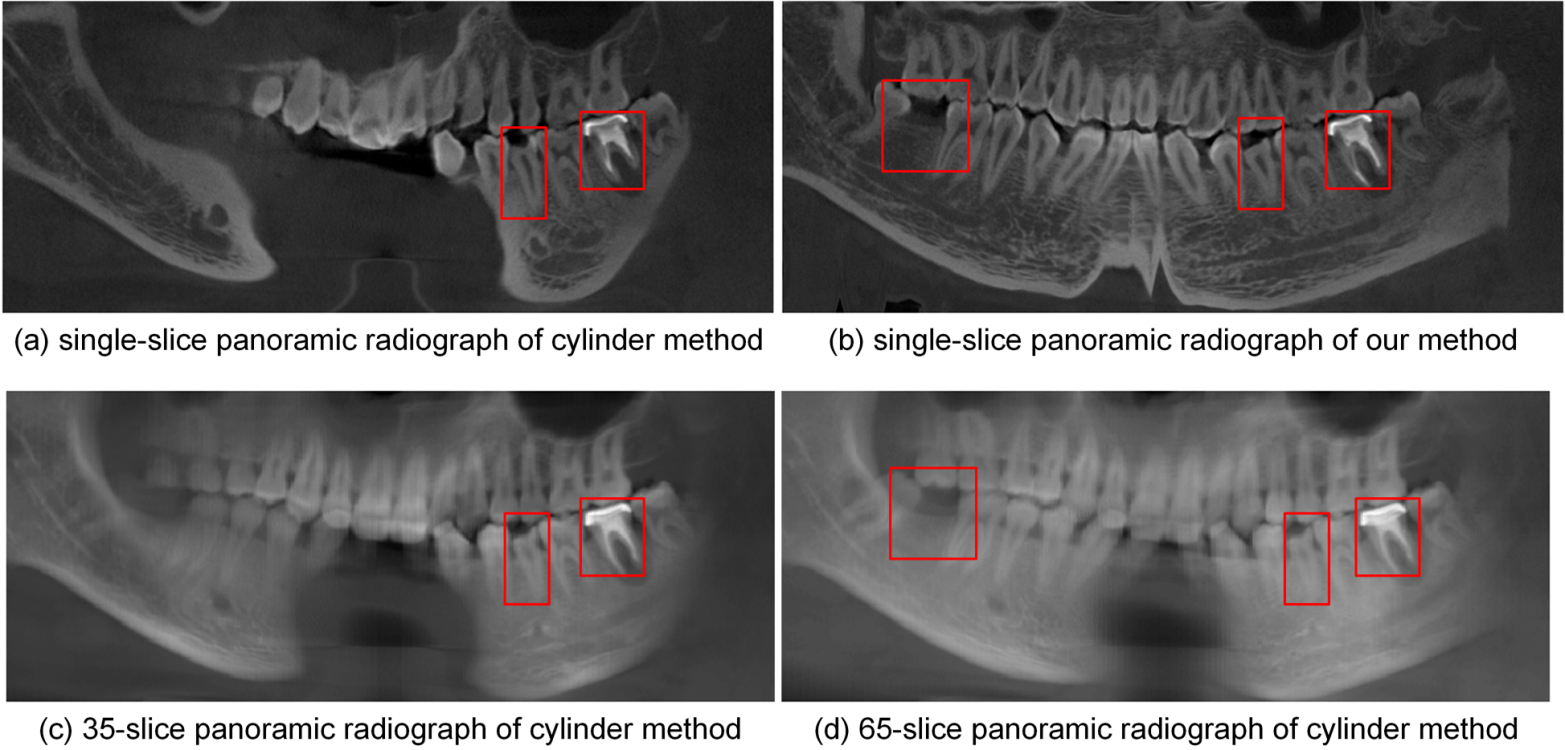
Panoramic radiographs of different methods from dataset 4.
